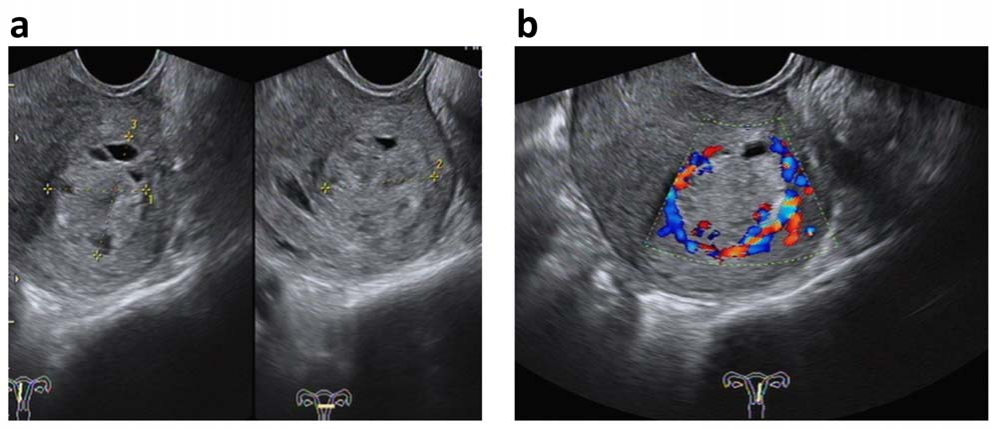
Ultrasonographic characteristics of ETT. (a) On gray-scale image, lesion was located in the left fundus of uterus, showing heterogeneously echogenic cystic-solid mass with distinct border (between cursors). (b) On Color Doppler, the abundant Doppler signals from blood flow distributed at the tumor periphery, while a few signals were showed within the boundary of tumor, which is named as “peripheral Doppler signal”.
